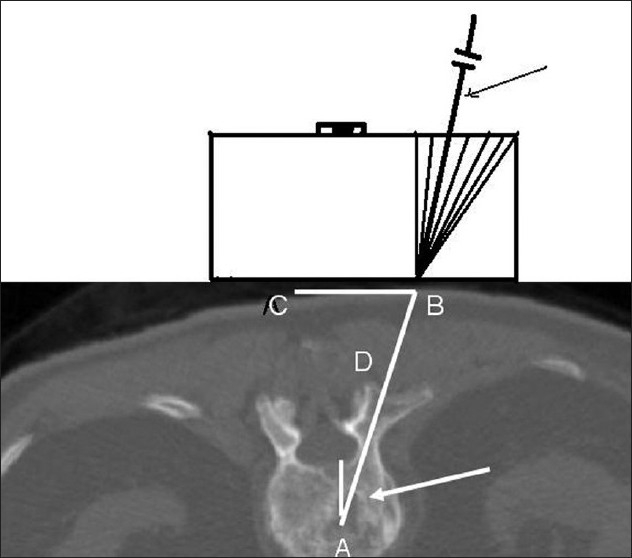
A sketch of the stereotactic guide positioned on CT axial image obtained during needle insertion. A-the target point; CB-distance from the midline to entry point of the needle on the skin surface; BD-distance from the skin surface to the top of the pedicle; BA- the distance of the trajectory from the skin surface to the target; and angle at A-angle of the trajectory with the vertical; white arrow-trajectory of the needle; black arrow shows the needle positioned in the appropriate groove in the stereo-guide. Note that the stereo-guide is held perfectly horizontal in the coronal plane
